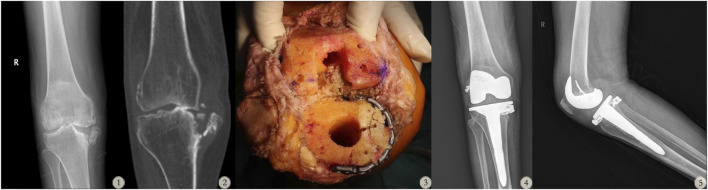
A 76-year-old female with Rand III type bone defect in the right knee. (1–2: Preoperative X-ray and CT scan of the patient’s right knee. 3: Intraoperative images showing the use of a tibial stem extender combined with a locking screw-plate to address the bone defect during TKA surgery. 4–5: Postoperative follow-up X-rays of the patient’s right knee.)
